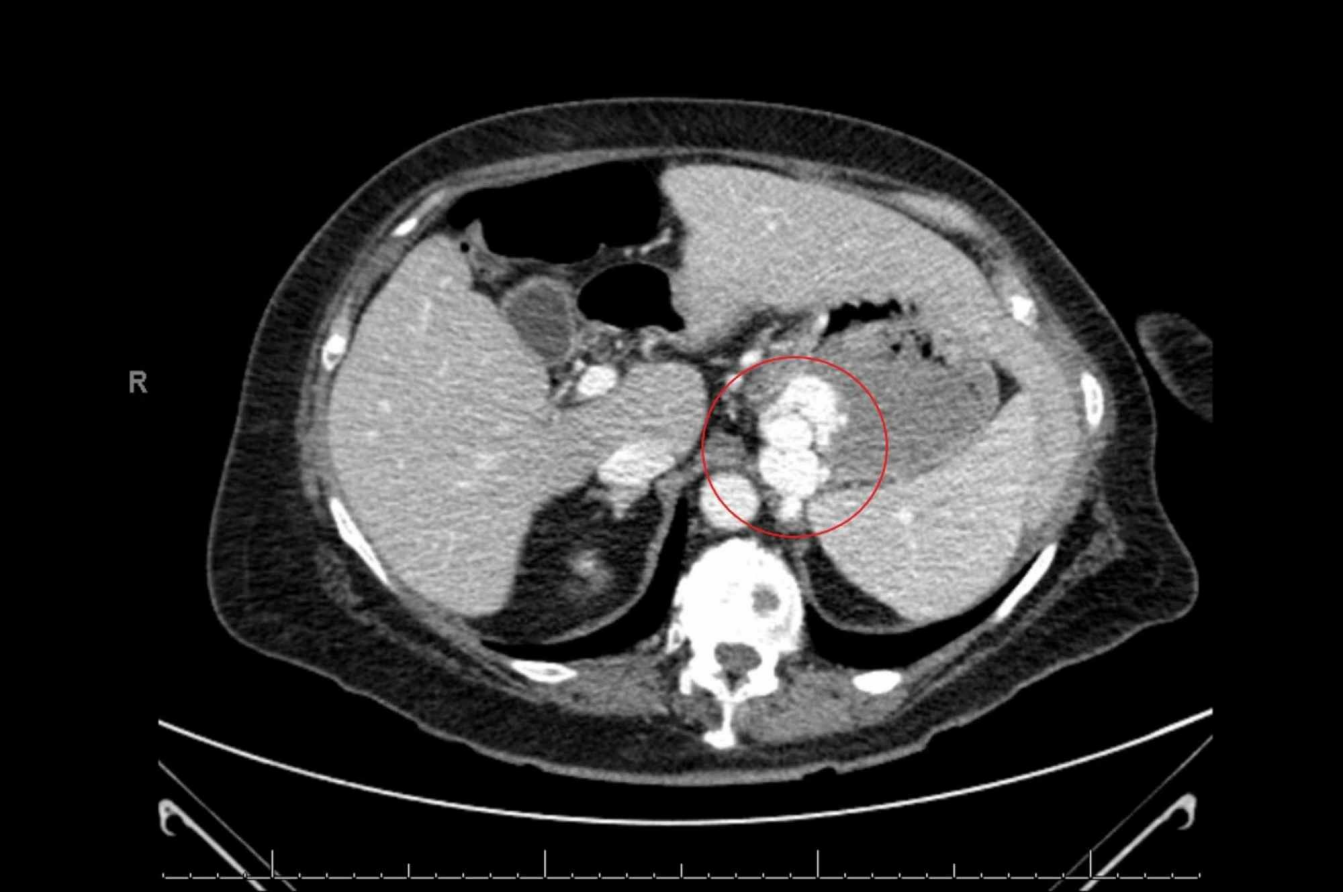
CT of abdomen/pelvis with IV oral contrast showing filling defect in the gastric cardia (highlighted)
